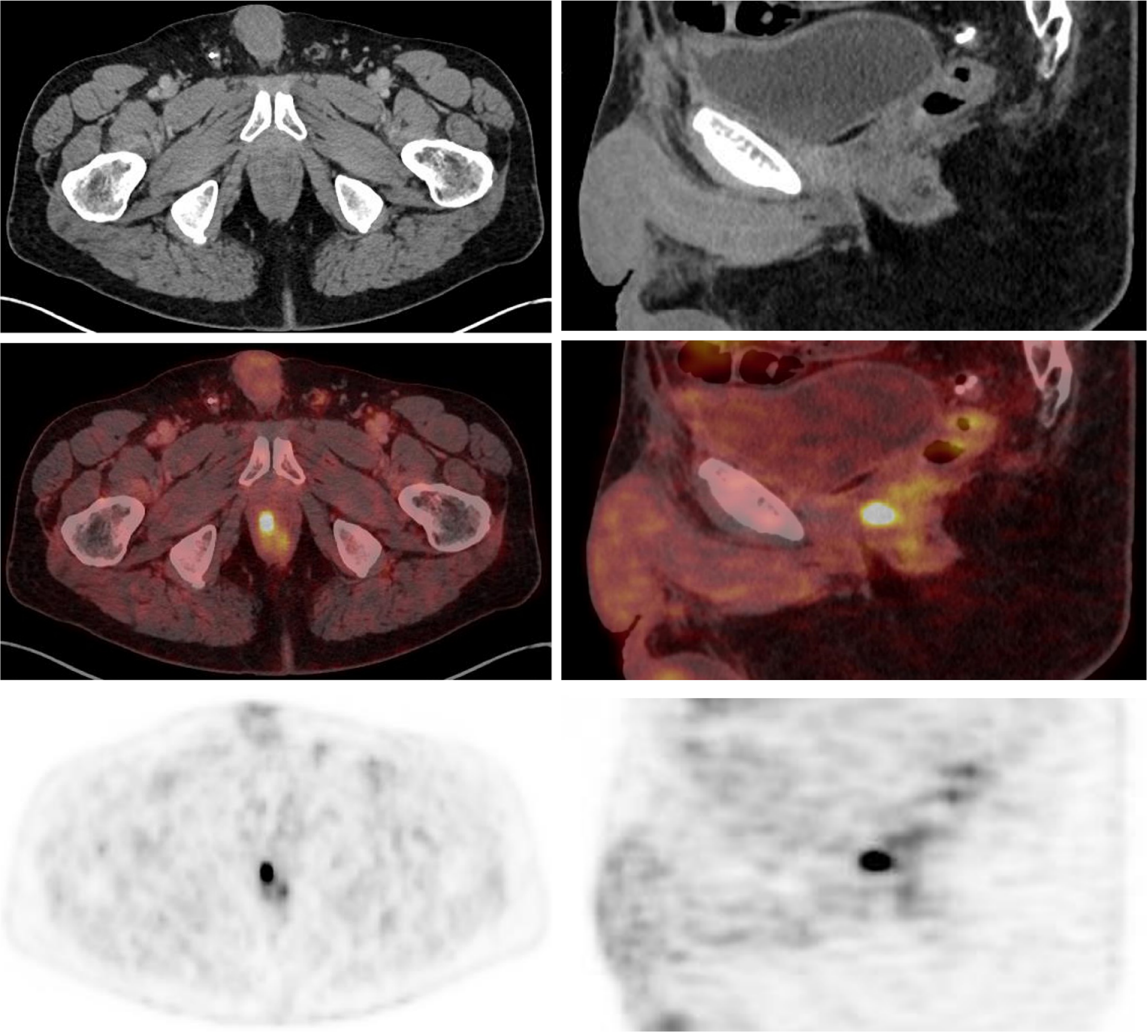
^18^F-PSMA-1007 PET/CT imaging (*left* axial images, *right* sagittal images) in a 72-year-old patient with biochemical relapse (PSA level 0.86 ng/ml) and with a Gleason score of 7b (4 + 3). The images show focal right-sided tracer uptake in the prostate bed
